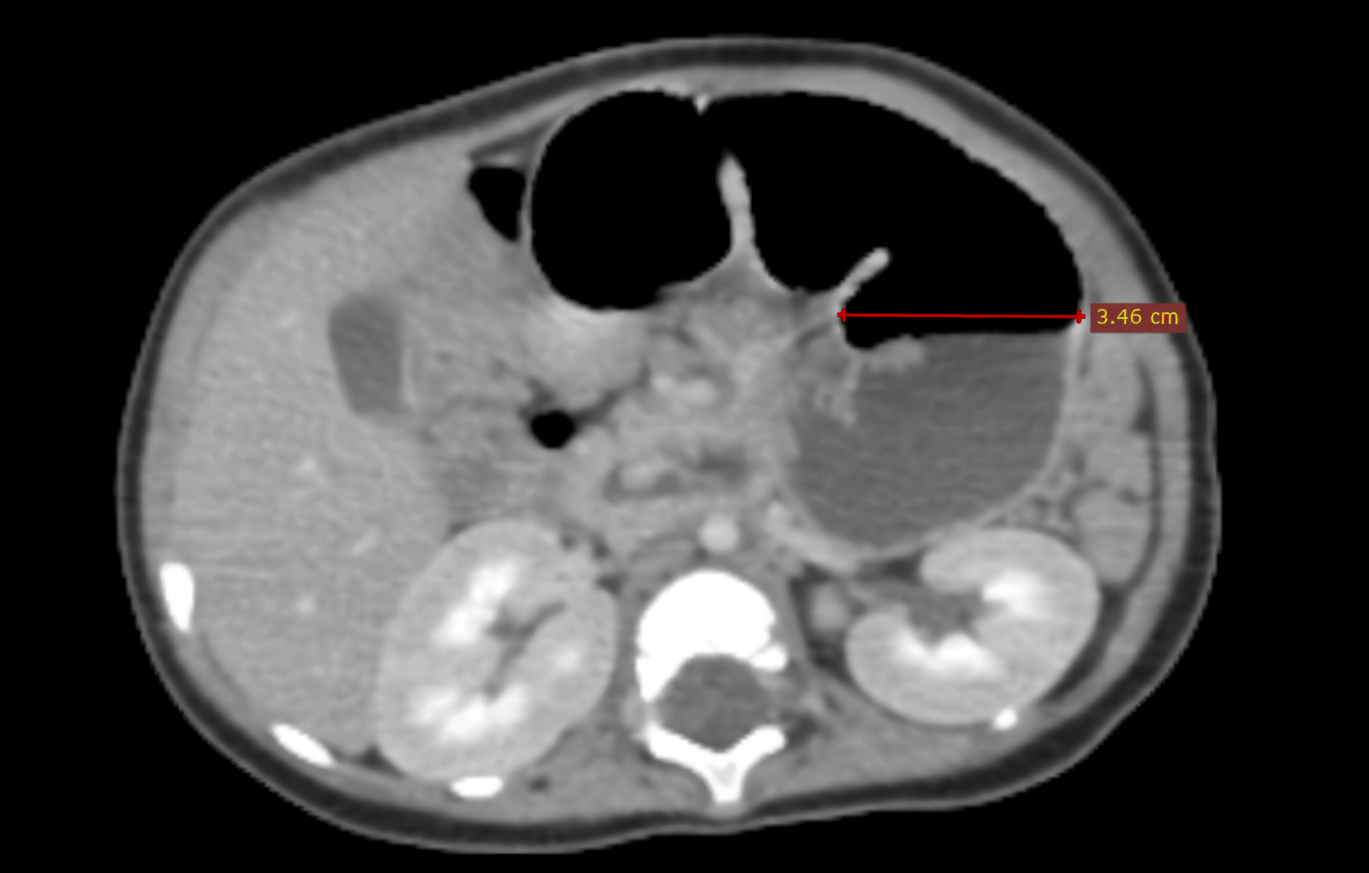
Axial CT image showing the distended loop prior to the obstruction transition zone
